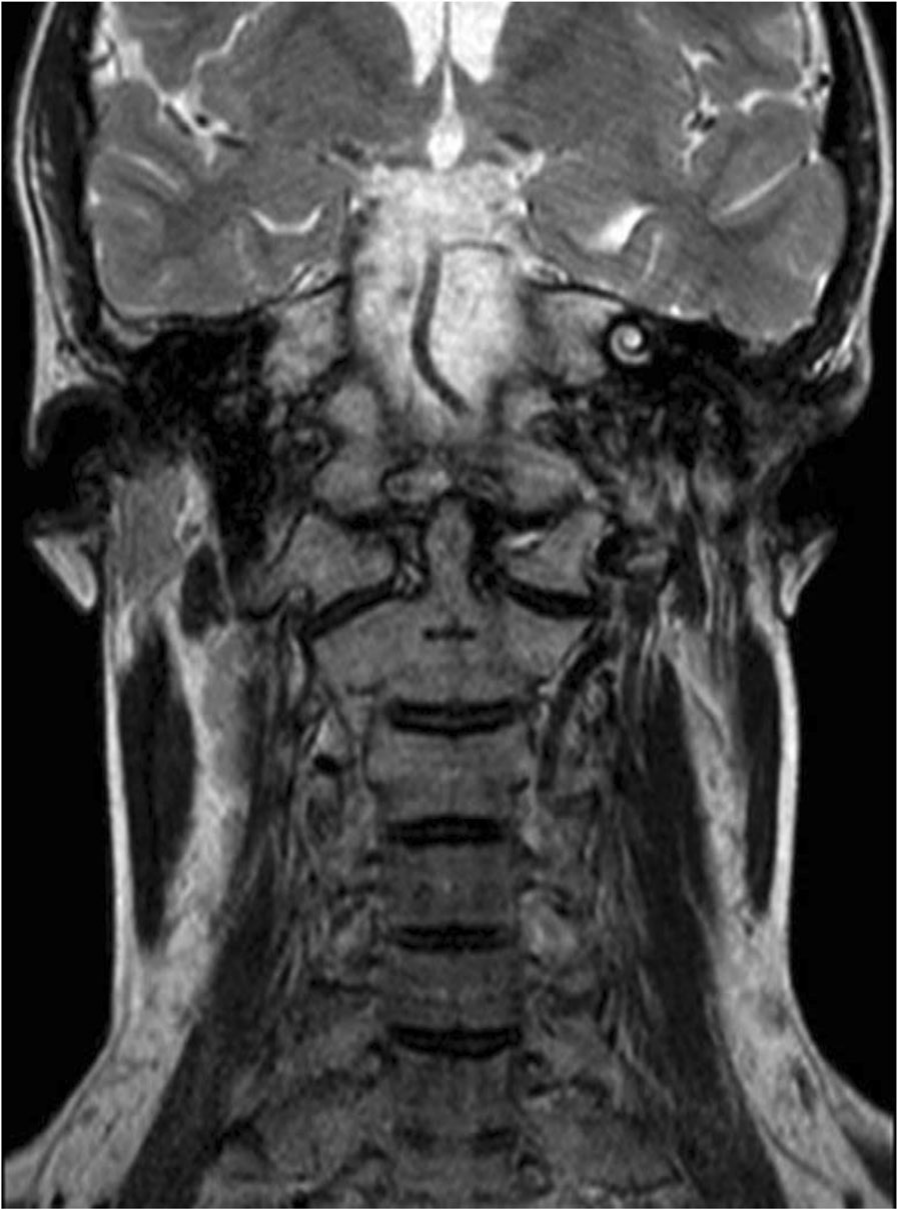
Non-homogenously edematous sternocleidomastoid muscles and infiltrated fat on the cervical spine MRI scan
